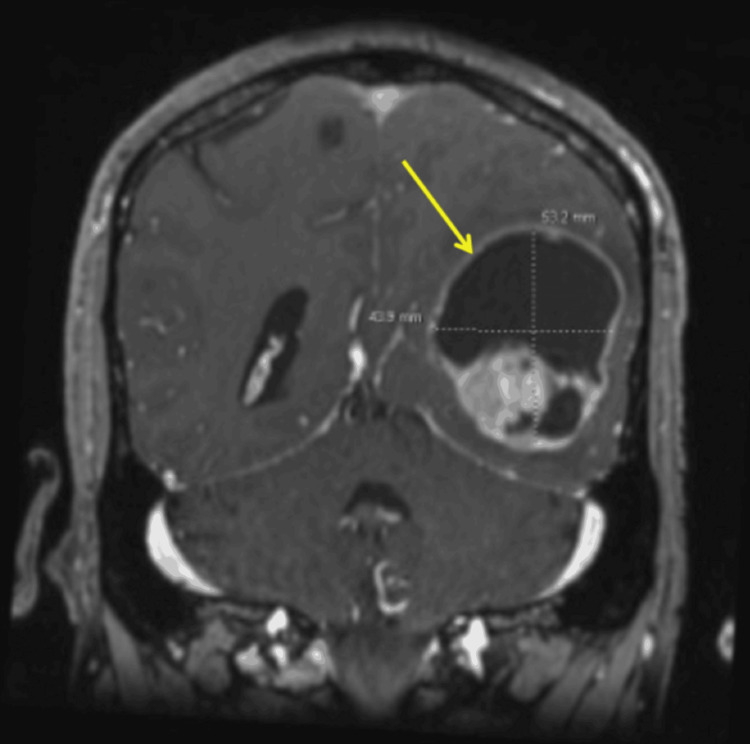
MRI of the brain revealed an enhancing, irregular mass with a large cystic component (5.3 x 4.3 cm, arrow) in the left parietotemporal lobe. MRI, magnetic resonance imaging
